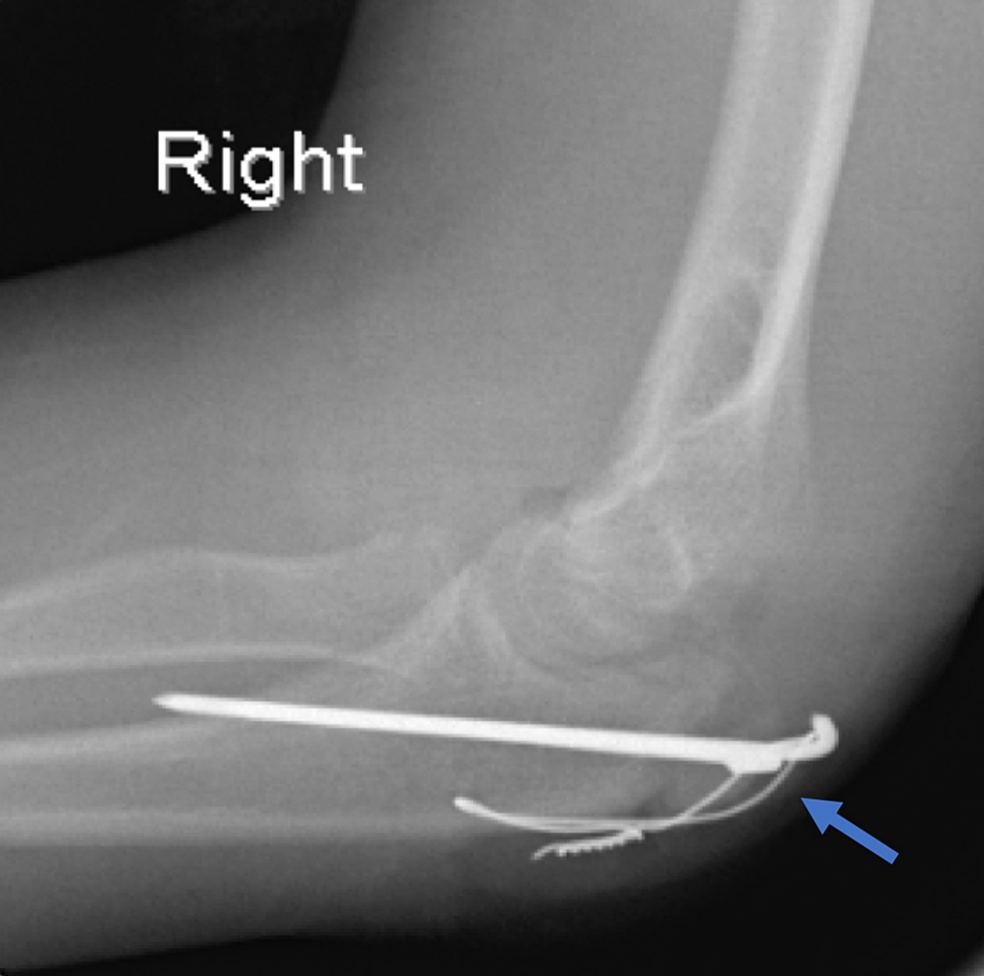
Lateral radiograph of the right elbow showing the tension band wire (arrow) technique with two 2 mm Steinman pins and an 18-gauge wire tension band.
